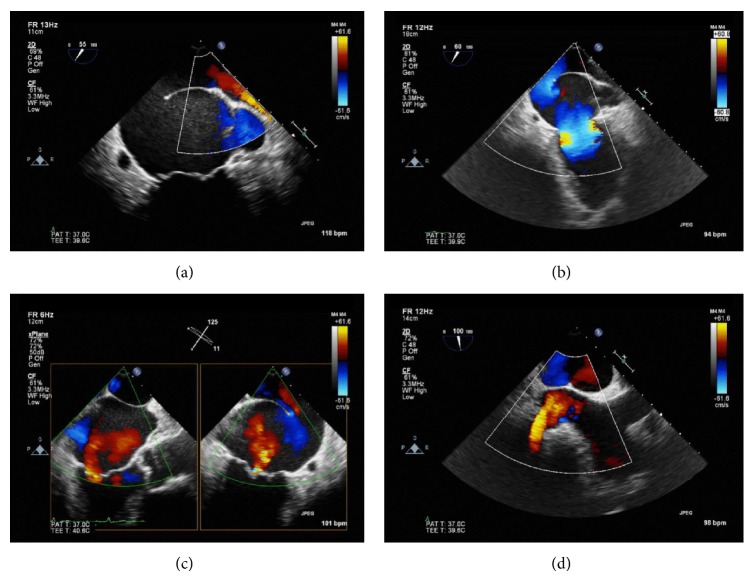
Transesophageal echocardiogram with Doppler color flow analysis. 2-chamber view showing flow from the pulmonary veins into the proximal accessory left atrium (a); flow across the fenestrated membrane into the distal true left atrium and across the mitral valve (b); biplane view showing mitral regurgitation and bidirectional flow across the membrane (c); and bicaval view showing partial attachment of the membrane to the interatrial septum (d).
